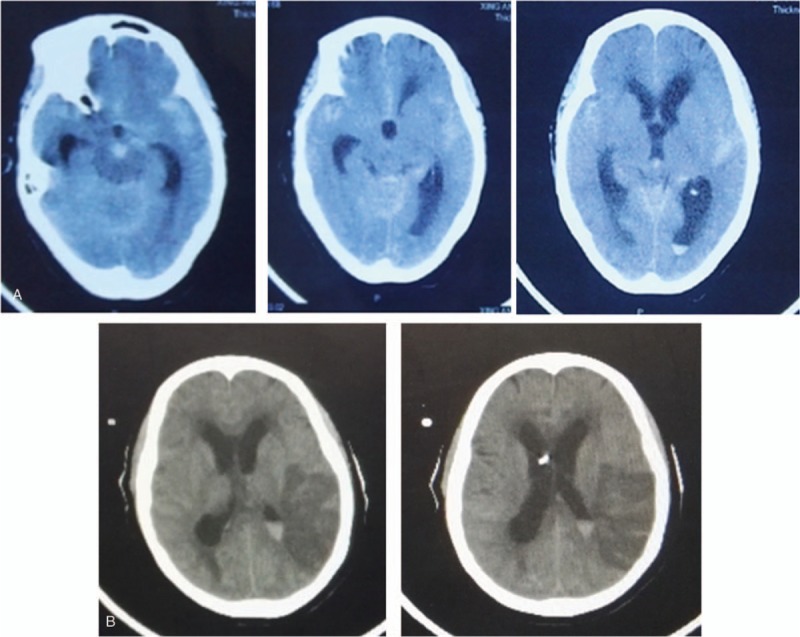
Emergency CT examination of the patient after 3 days of cerebral angiography. The images of CT showed hydrocephalus (A), an emergency external ventricular drainage was performed. Postoperative CT scan showed cerebral infarction in the left temporal lobe (B). CT = computed tomography.
